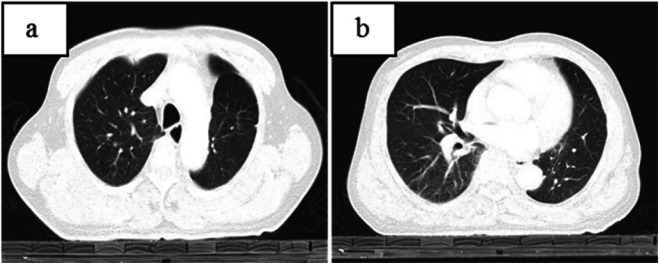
The chest CT showed that two corresponding nodules in the original right lung had disappeared after 4 years later
